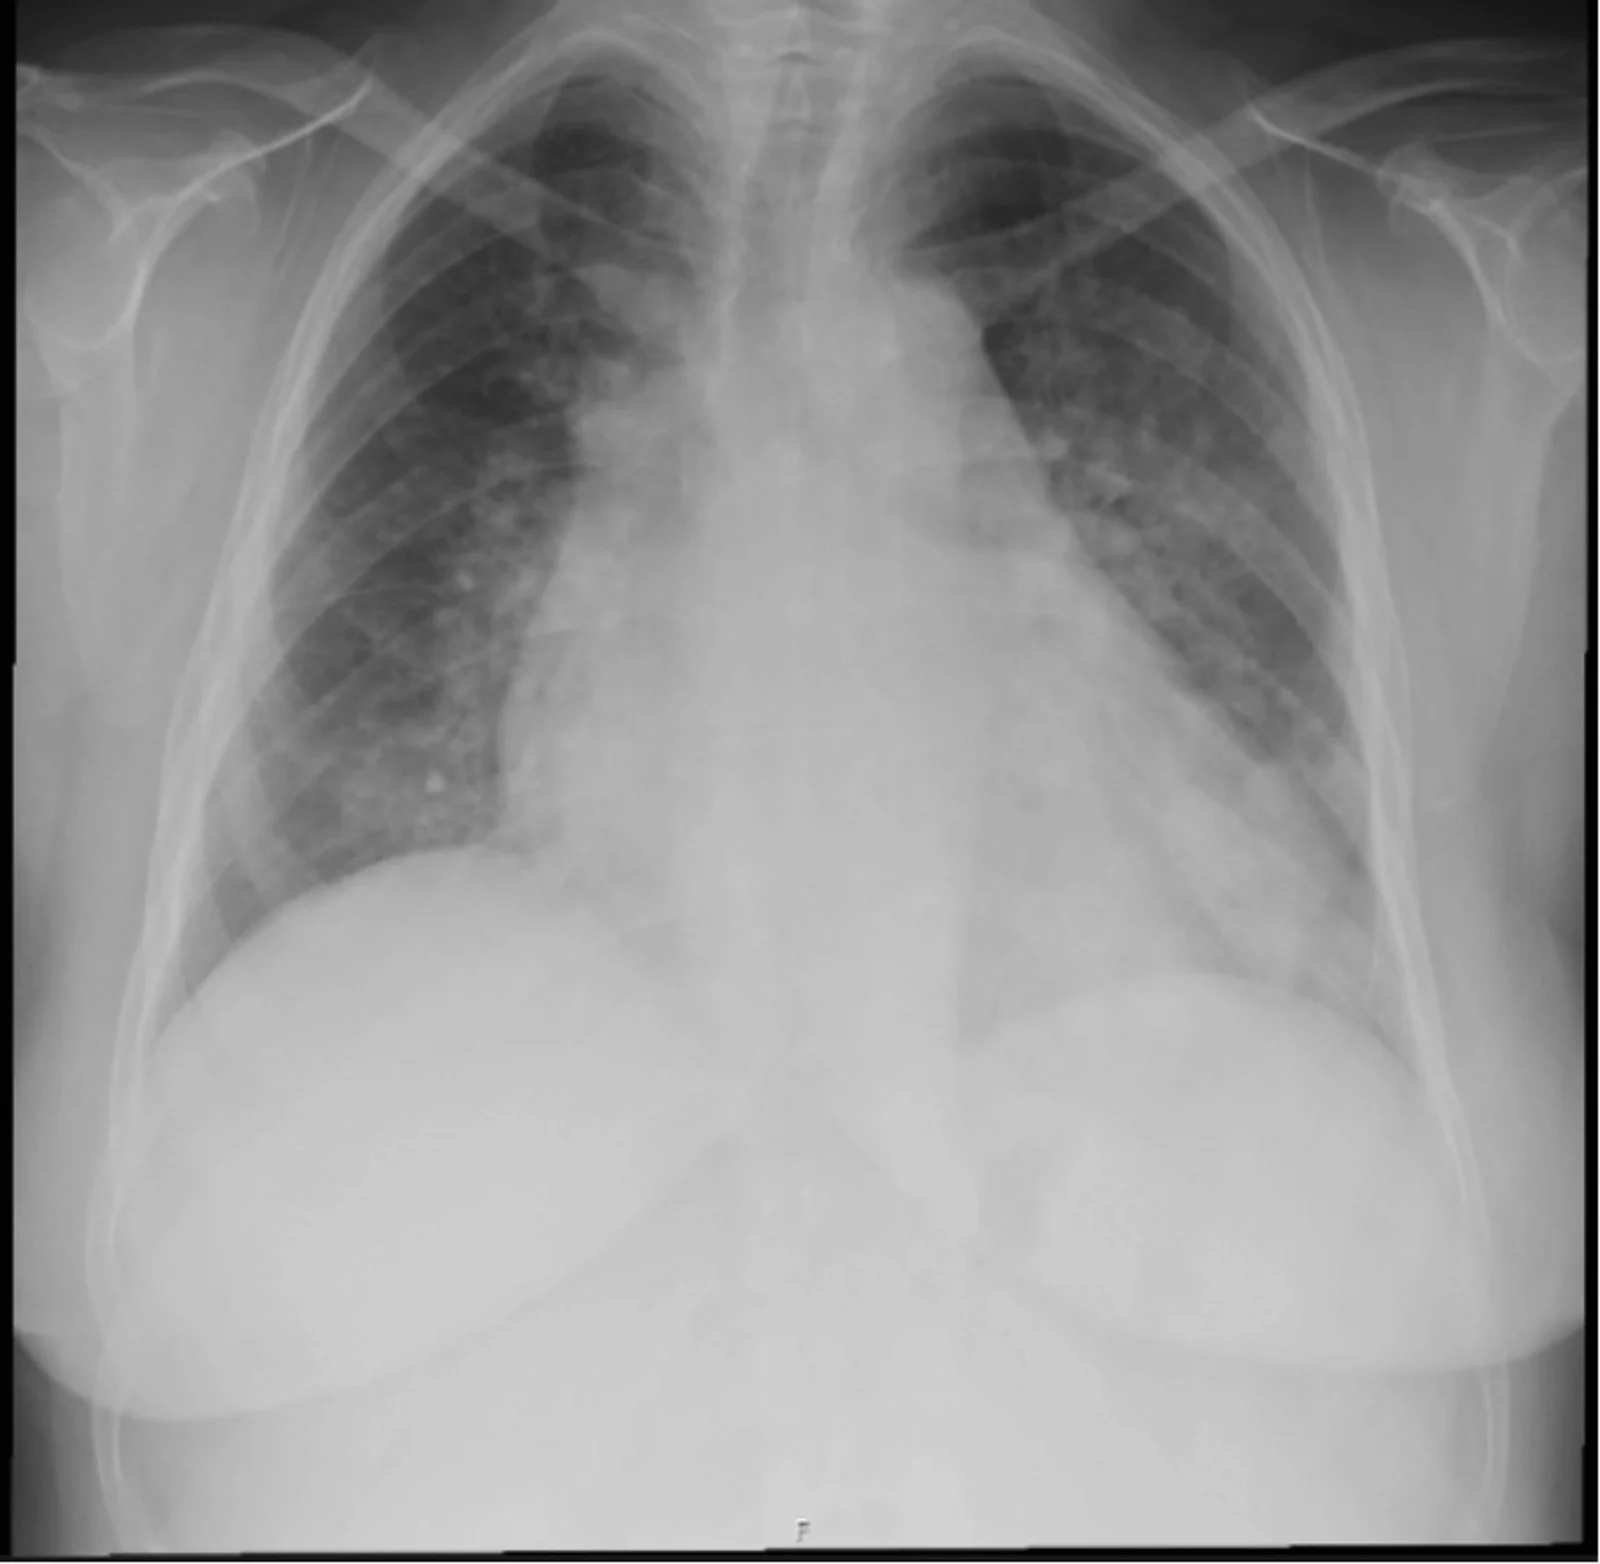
Chest X-ray performed on admission, showing no evident consolidations.
